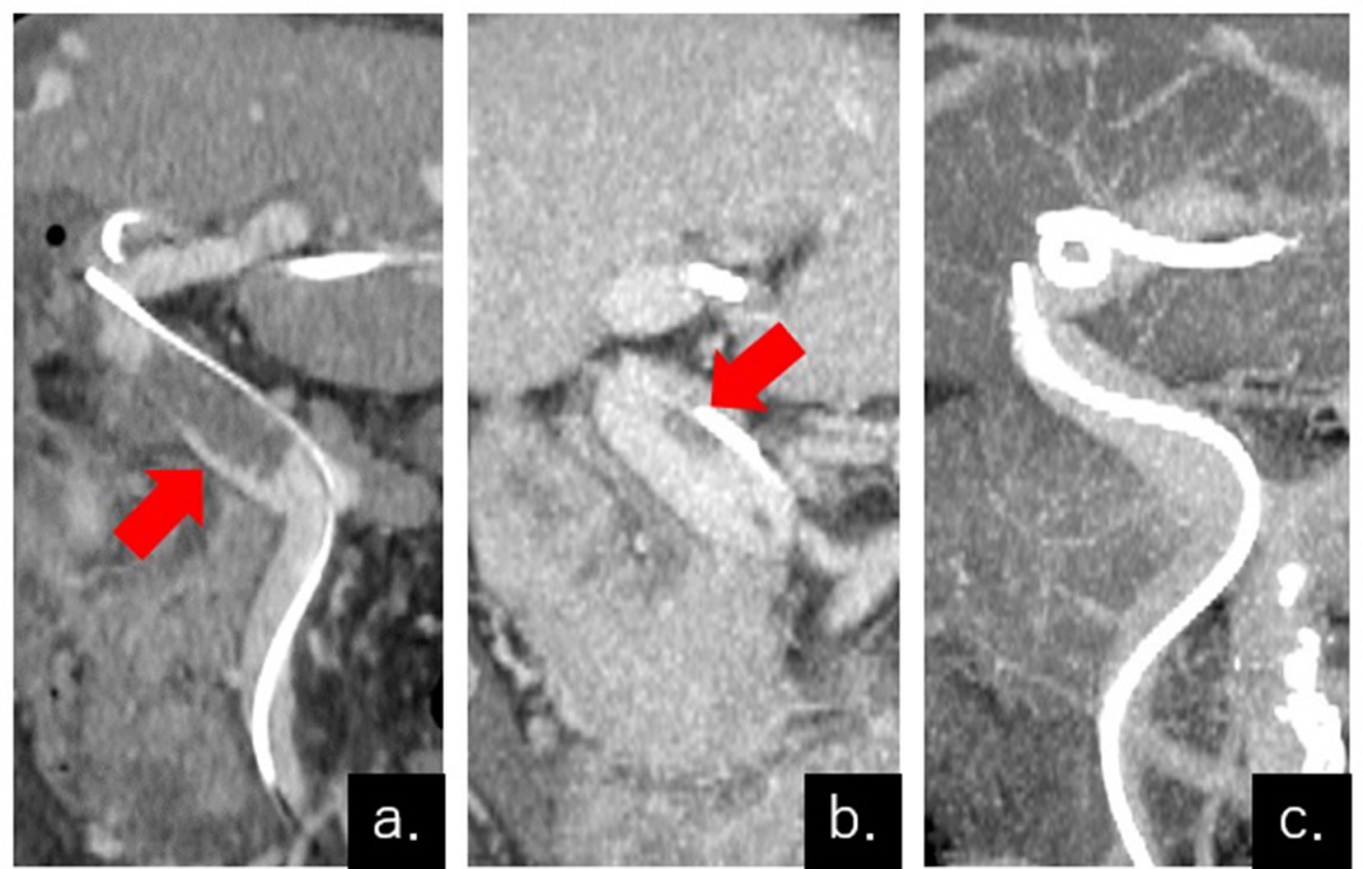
Comparison of the portal vein observed by CECT before reoperation and by fluorography during reoperation. (a) CECT image of the “defective part” shown in the main portal vein trunk indicating the thrombus (arrows). (b) Digital subtraction angiography image after insertion of a Destination™ guiding catheter. The “defective area” was in the same place as in the CECT image in (a) (arrows). (c) Thrombectomy and aspiration were performed using a Vasplyser Plus™ thrombus aspiration catheter and the portal vein was recanalized (arrows). CECT, contrast-enhanced CT.
